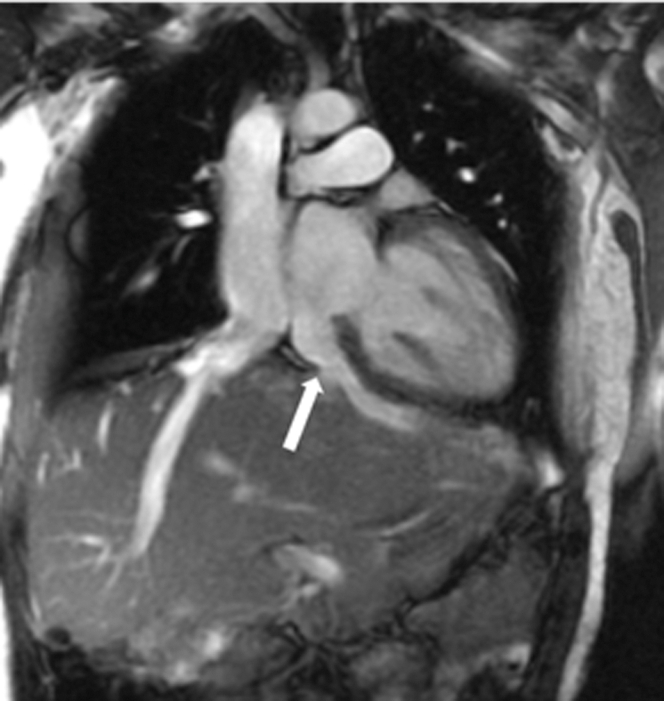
Anomalous Left Hepatic Vein to Left Atrium Magnetic resonance imaging showing a midline liver with left hepatic vein draining directly to the left atrium (arrow) and a right hepatic vein connecting to the suprahepatic inferior vena cava that eventually drains to the right atrium.
